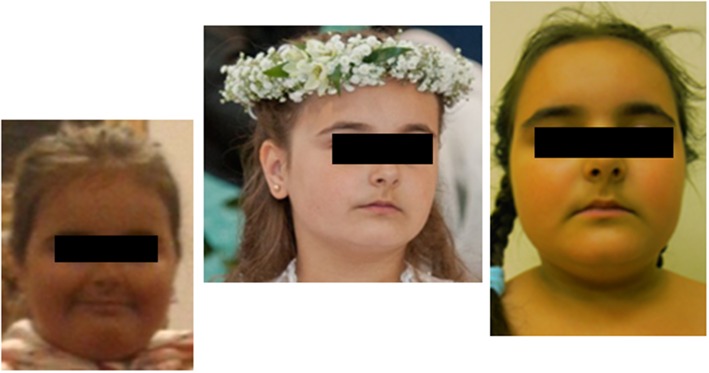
MR image of the hypothalamic-pituitary area with contrast with visible pituitary adenoma in the patient with cyclic Cushing's disease in the second relapse period (clinical and biochemical hypercortisolemia at the age of 8 6/12 years).
